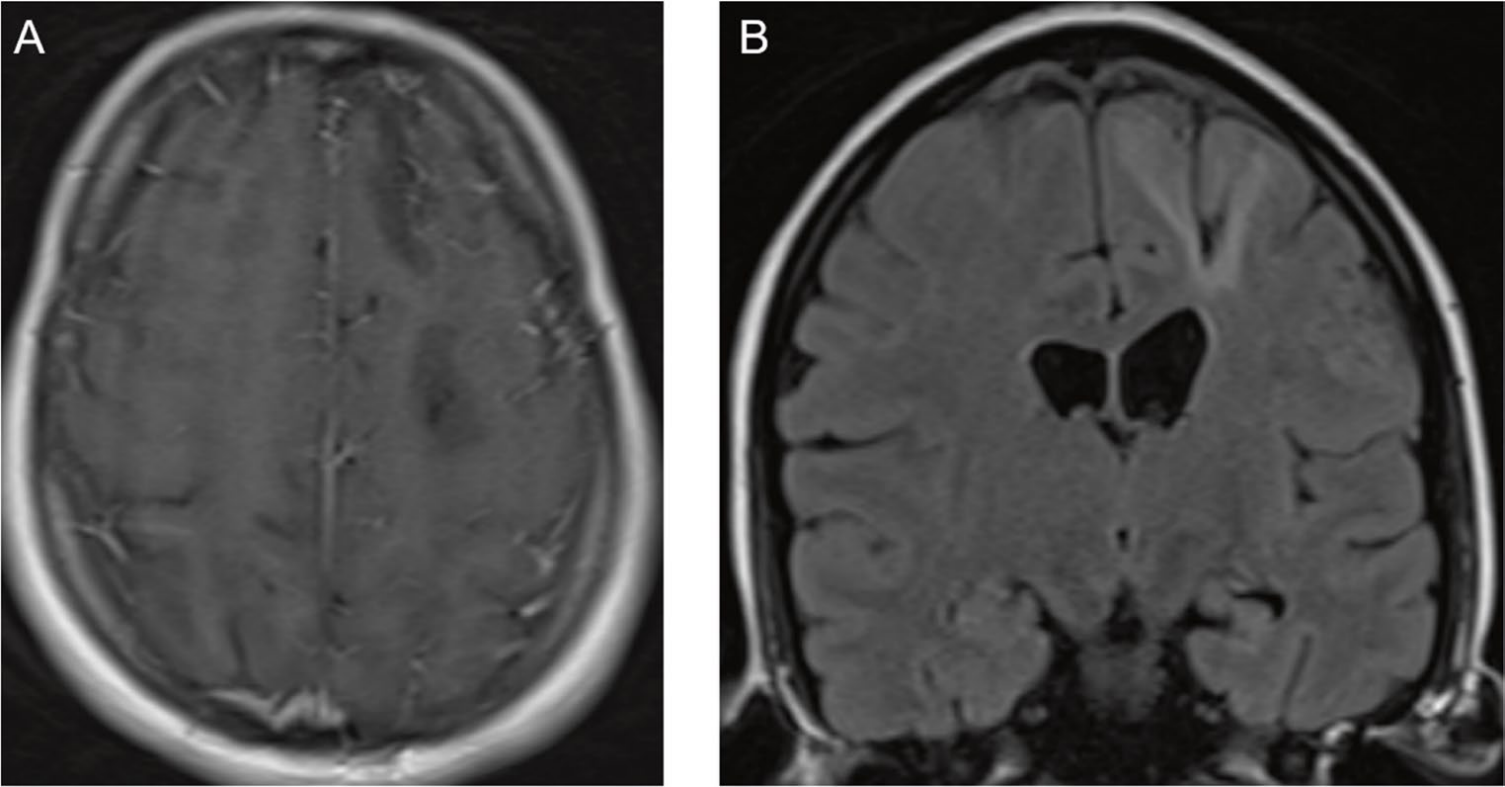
(A) Axial post‑contrast T1‑weighted MRI after corticosteroid therapy showing near‑complete regression of the previously observed leptomeningeal enhancement. (B) Coronal non‑contrast FLAIR demonstrating residual cortical/subcortical sequelae predominantly in the right superior and middle frontal gyri. Abbreviations: MRI = magnetic resonance imaging; FLAIR = fluid‑attenuated inversion recovery
